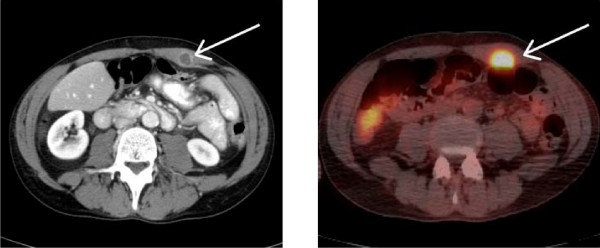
Imaging of left rectus abdominus mass. A.) CT scan performed during ED visit demonstrating a 14 mm lesion in the left rectus muscle. B.) Subsequent PET-CT demonstrated significant 18-FDG uptake at the site of the lesion found on CT. No other sites of abnormal uptake were found.
